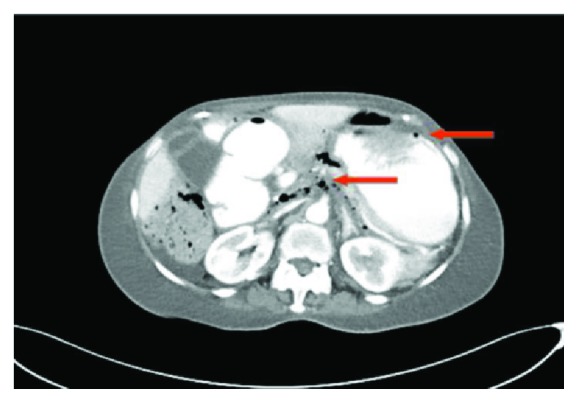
CT scan of the abdomen. Retroperitoneal free air.
